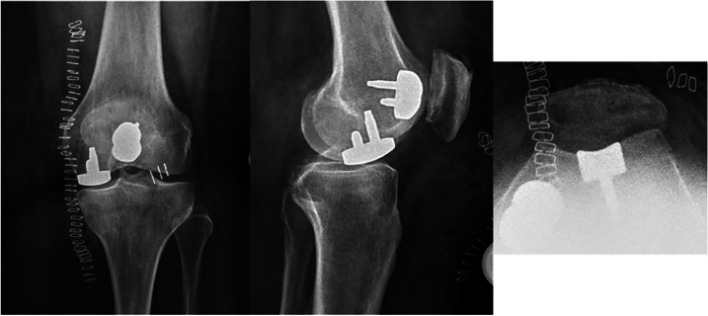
Postoperative x-ray after implantation of two patient-specific implants
